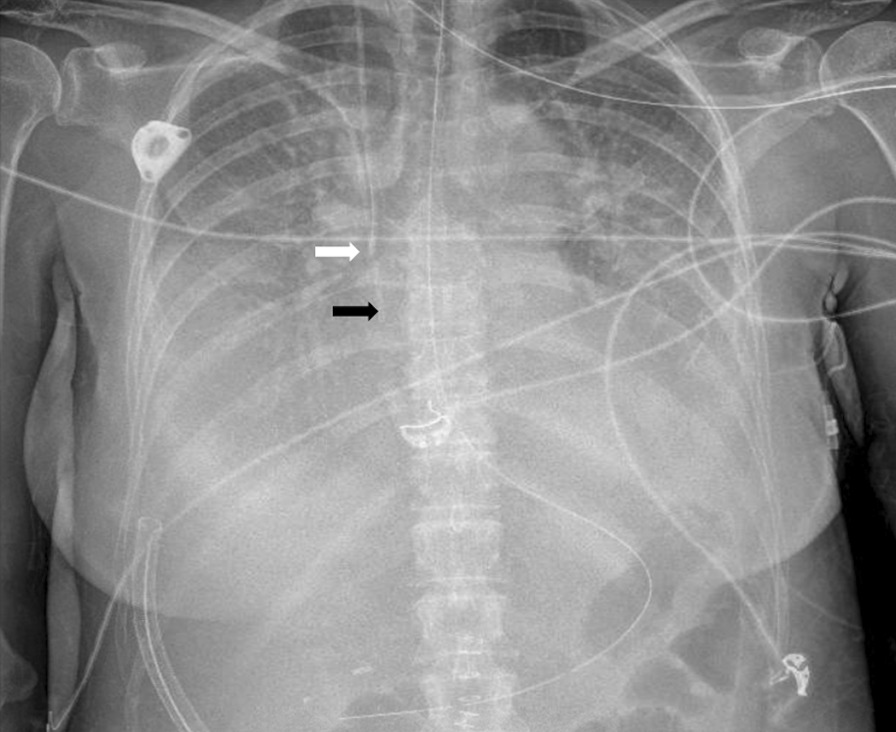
Chest x-ray examination showing the correct position of the tip of the Port-a-Cath (white arrow) and central venous (black arrow) catheter in the left internal jugular vein. Large bilateral pleural effusions are seen.
